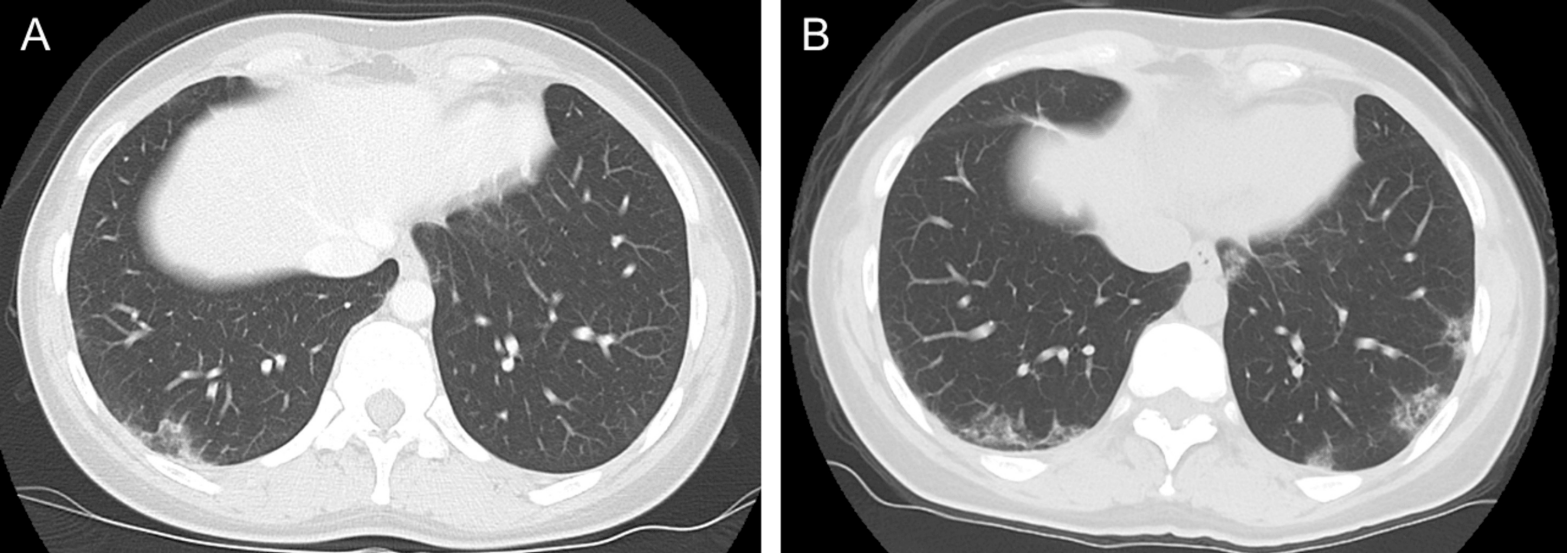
Chest computed tomography of the second case (A) Ground-glass opacity only in the base of the right lung. (B) Multiple ground-glass opacities in bilateral lung bases on recurrence.
